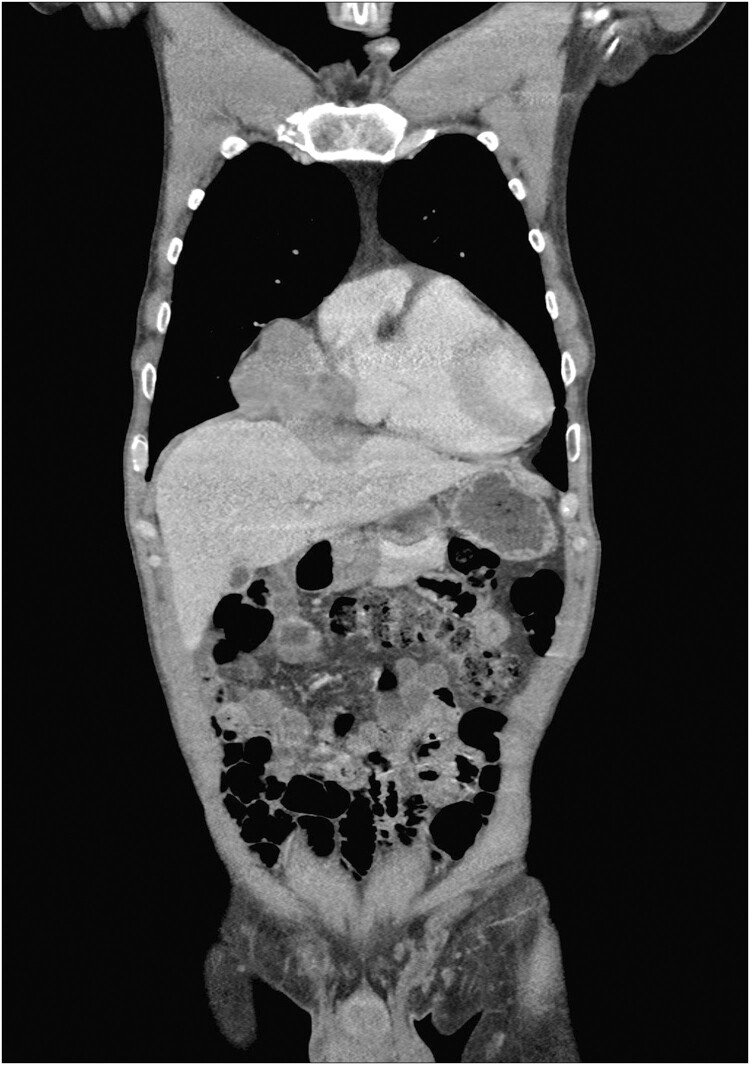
Computed tomography scan showed an 8 × 6 × 9 cm tumorous process along the right side of the heart.
